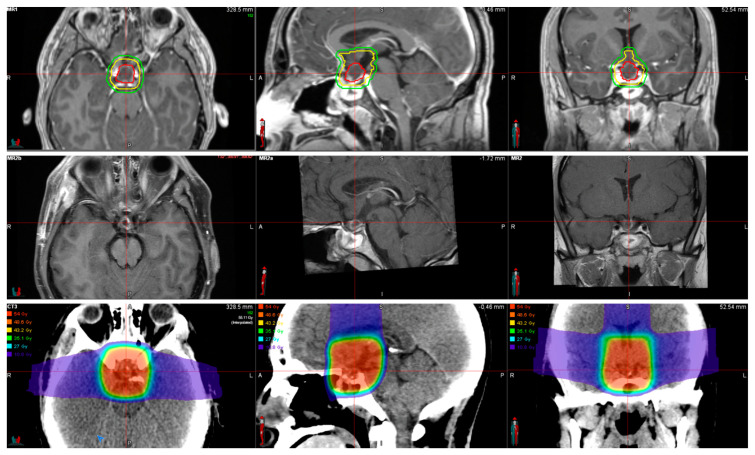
Twenty-nine year old patient with recurrent adamantinomatous craniopharyngioma treated with salvage surgery and post-operative proton radiotherapy. Row 1. Pre-op MRI shows suprasellar cystic tumor with enhancing wall involving the optic pathway. Pre- and post-operative tumor volumes inform the radiation target contours as overlayed on the images. GTV (red) = gross tumor volume (based on pre-op gross tumor), CTV (yellow) = clinical target volume (encompassing areas of tumor extent at initial presentation and areas at risk of subclinical disease), PTV (green) = planning target volume. Row 2. Post-op MRI shows residual cystic tumor involving the optic pathway. Row 3. Radiation planning CT with radiation dose overlay. Pencil beam scanning proton radiation was utilized to deliver a prescription dose of 54 GyRBE in 1.8 GyRBE fractions.
